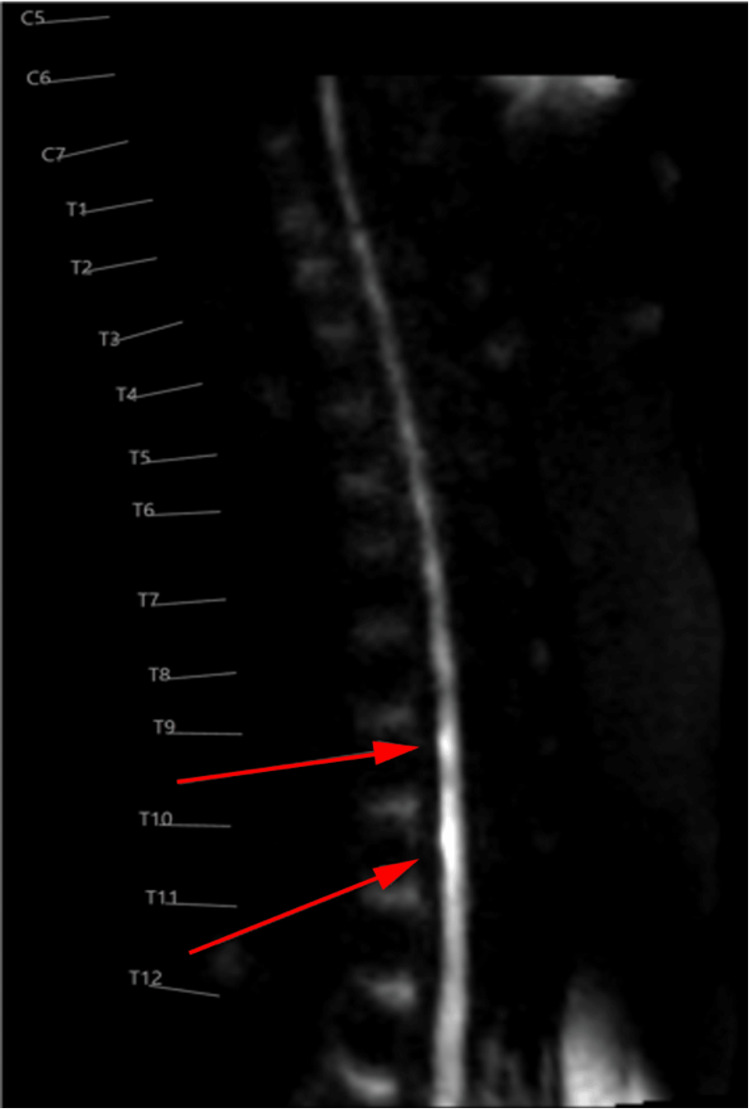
Sagittal DWI of the thoracic spine demonstrating diffusion restriction at the site of the signal abnormality from T8 to T9 through T11 to T12, indicated by red arrows DWI, diffusion-weighted imaging
